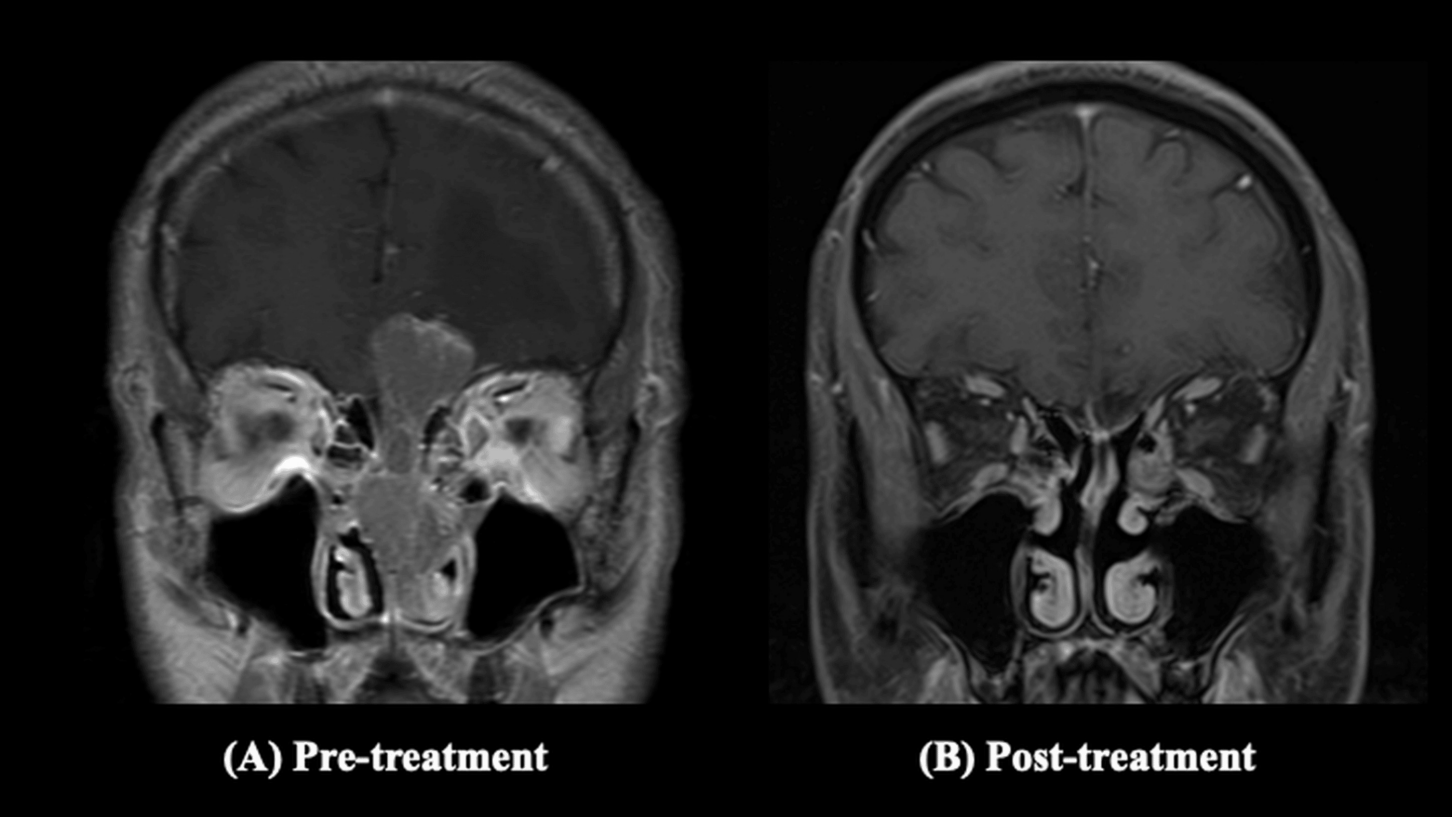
Coronal gadolinium-enhanced T1-weighted magnetic resonance images before and after treatment (A) Pre-treatment MRI shows a strongly enhancing mass occupying the left nasal cavity with superior extension into the anterior cranial base; (B) Post-treatment image demonstrates complete resolution of the enhancing lesion following concurrent chemoradiotherapy.
